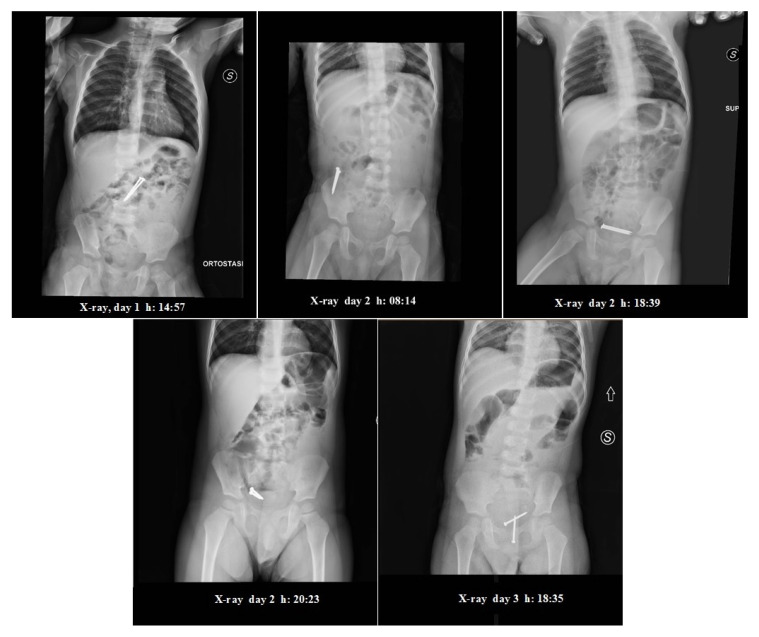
Serial abdominal X-rays.
